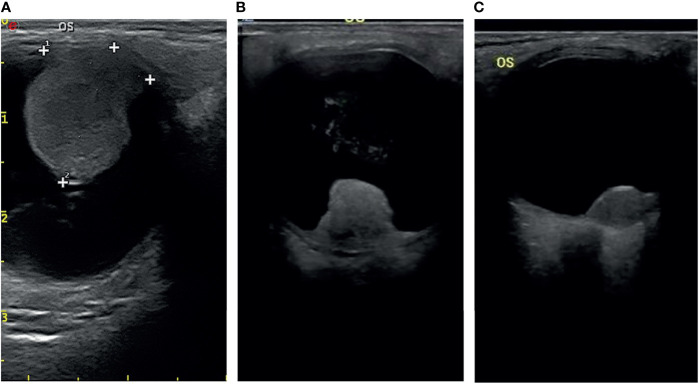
The typical pattern of UM in B-scan ultrasonography (A), mushroom configuration (B), and acoustic hollowness (C) choroidal excavation.
